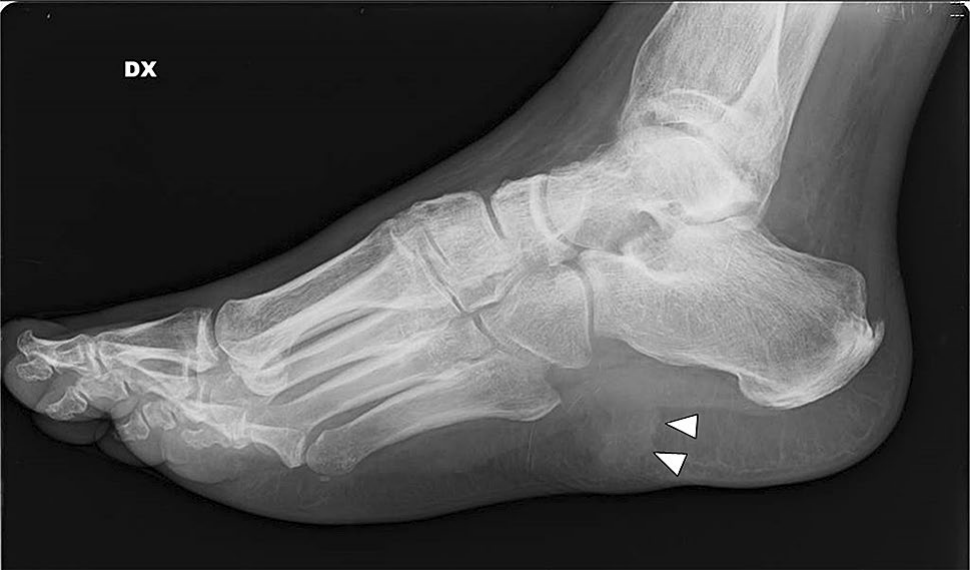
44-year-old man with a PMT of the foot. Plain radiograph demonstrates a faint increased density in keeping with soft tissue calcification on the plantar aspect of the foot
